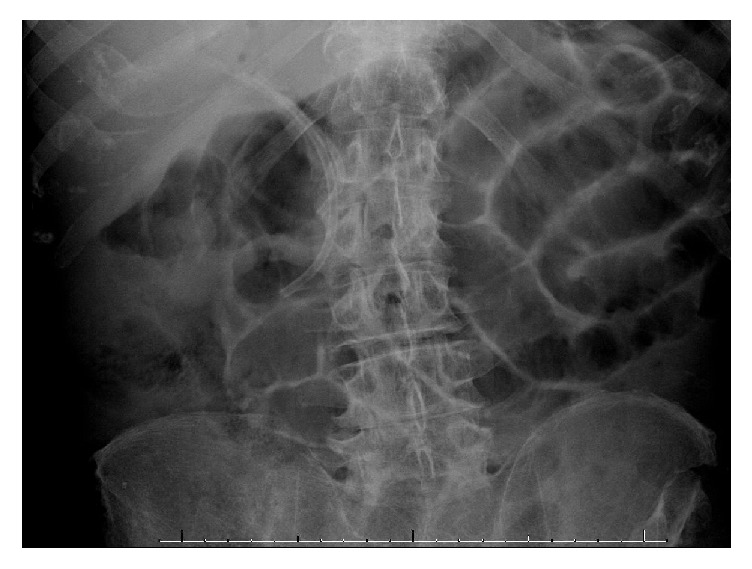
Abdominal radiograph showing appropriate position of the new plastic stent. The old, migrated stent was left inside the bile duct.
